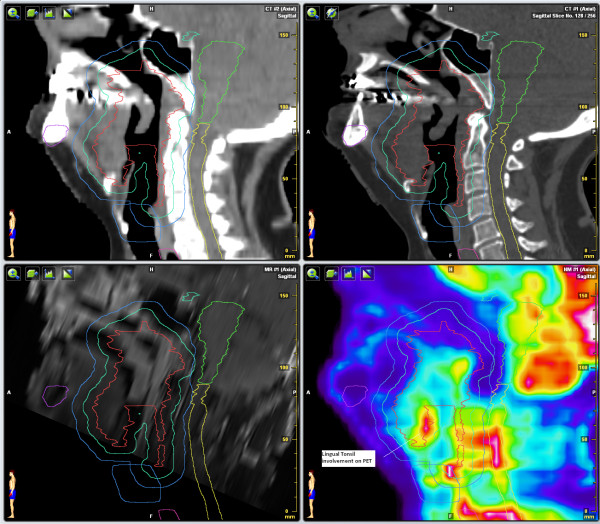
CT (soft tissue windows and bone windows), MRI, and PET sagittal views. Lingular tonsillar involvement is visualized on the PET, but was not detected using CT or MRI.
